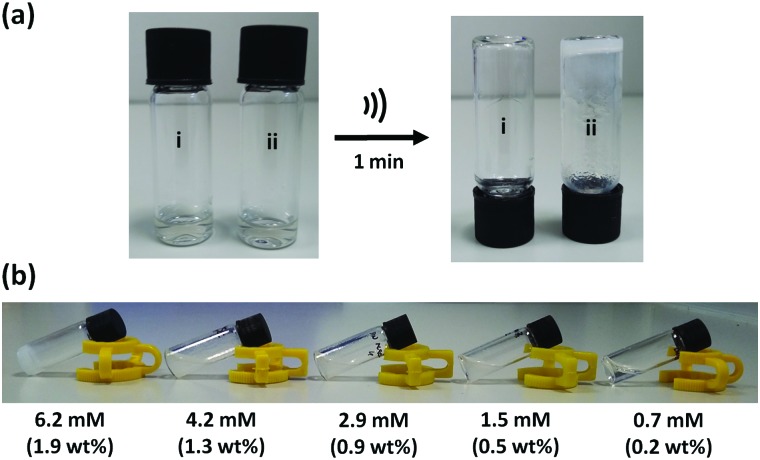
(a) Ultrasound-induced gelation of compound 1 in CH_3_CN at 14 mM. The left image shows two identical solutions of 1. The right image corresponds to the sonication of solution (ii) for 1 min and solution (i) is the negative control. (b) Gel formed upon sonication of solutions of 1 in CH_3_CN at different concentrations.
